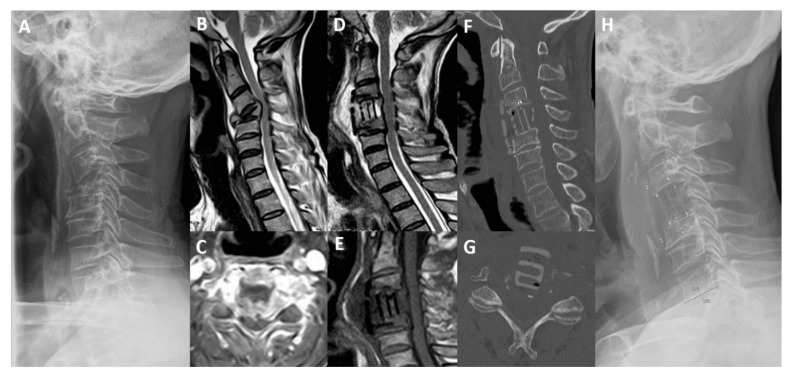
Magnetic resonance imaging (MRI), computed tomography (CT) and standing lateral radiograph of cervical spine. (A) Preoperative standing cervical lateral X-ray. (B) T2-weighted sagittal MRI. (C) T1-weighted with gadolinium axial MRI. (D) Post-operative T2-weighted sagittal MRI. (E) Post-operative T1-weighted sagittal MRI. (F) Post-operative (3 months) sagittal CT scan. (G) Post-operative (3 months) axial CT scan. (H) Post-operative standing cervical lateral X-Ray, showing a pathological fracture of C4 vertebra in a 59-year-old man with lung cancer and intractable neck pain and myelopathy. He underwent anterior C4 corpectomy and plate fixation with carbon-PEEK implants with resolution of pain and improvement of Nurick scale from grade 3 to 0. (TS = 9; SINS = 13).
